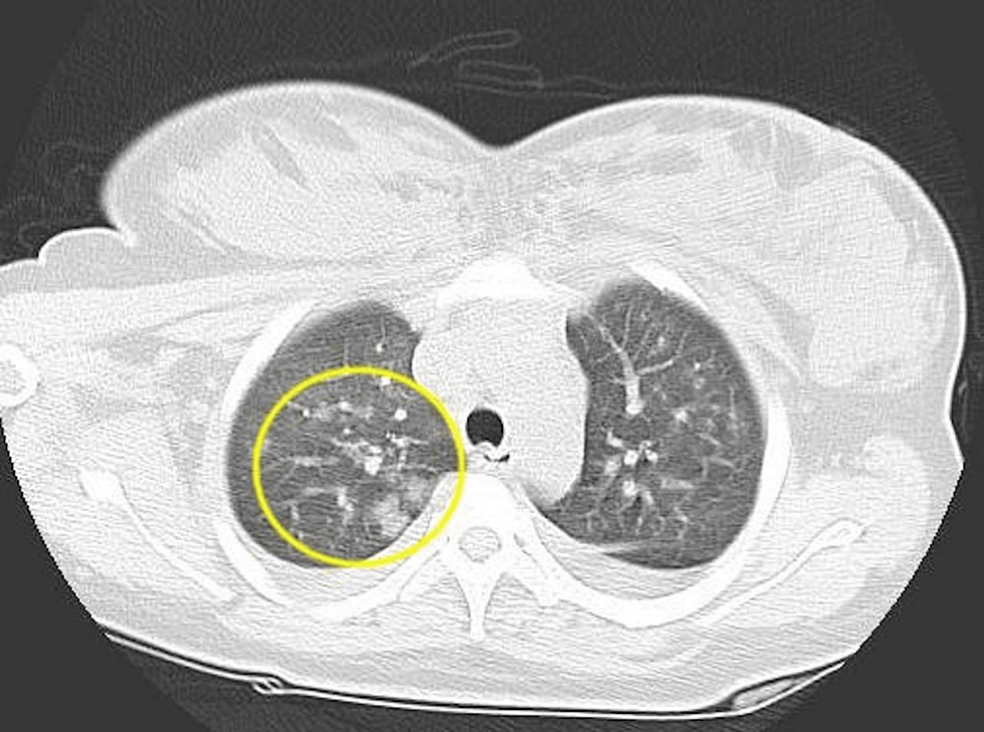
CT image of the chest Frosted shadows in the right upper lung field (yellow circle)
